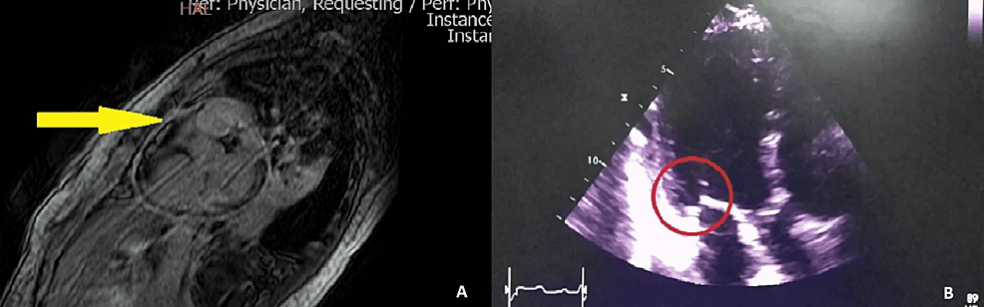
Cardiac magnetic resonance and transthoracic echocardiogram Panel A shows that the two ventricles are dilated, hypokinetic, with severe systolic dysfunction of the left ventricle. Pericardial thickening is observed adjacent to the mid-apical inferolateral segments of 3mm with enhancement after injecting contrast medium. Myocarditis is established by the Lake Louise criteria showing areas of high signal intensity in a non-ischemic distribution pattern on late gadolinium enhancement images in T1 and high signal intensity of the pericardium consistent with pericardial inflammation. Panel B shows an echodense, sessile mass, adhered to the posterior wall of the left atrium 7mm from the mitral annulus, compatible with vegetation.
